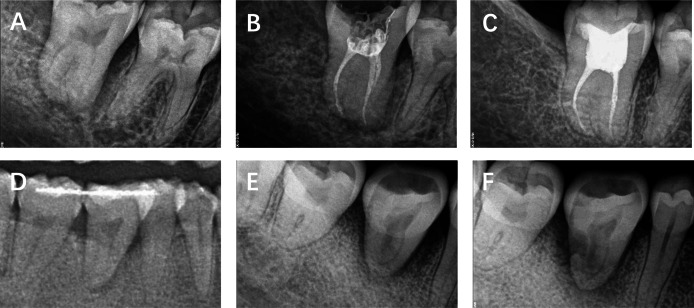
The radiographic images of representative cases. (A–C) Case 1. (A) Immediately postoperative. (B) Root filling completion at postoperative 4 weeks. (C) Follow-up at postoperative 24 months. (D and E) Case 2. (D) Immediately postoperative. (E) Rejecting RCT treatment at postoperative 8 weeks. (F) Follow-up at postoperative 28 months.
